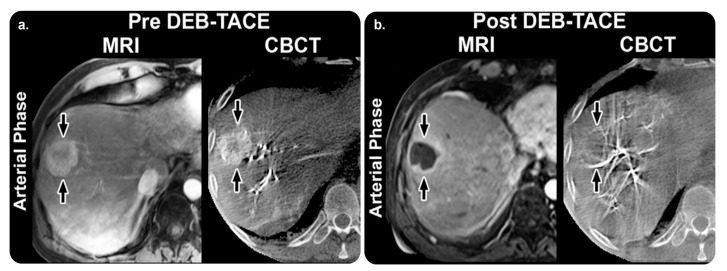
SmartCT software soft tissue technique and its role in management planning. (a) Arterial-phase images obtained before TACE. MRI revealing a 75 mm mass in the right lobe “arrow” with 65% enhancement. The mass has a similar enhancement (75%) with CBCT. (b) Arterial-phase images obtained after TACE. Mass shows almost no enhancement (5%) with MRI, and the tumor is decreased to 69 mm. With CBCT, the intraprocedural mass enhancement is decreased by 87%, which allows prediction of an objective EASL response at 1 month. Abbreviations: TACE, transarterial chemoembolization; MRI, magnetic resonance imaging; CBCT, cone-beam computed tomography; EASL, European Association for the Study of the Liver. Reprinted from “Intraprocedural C-arm dual-phase cone-beam CT: Can it be used to predict short-term response to TACE with drug-eluting beads in patients with hepatocellular carcinoma?” by Loffroy et al. [14] with written approval from the corresponding author and publisher (Radiology; Copyright 2013).
